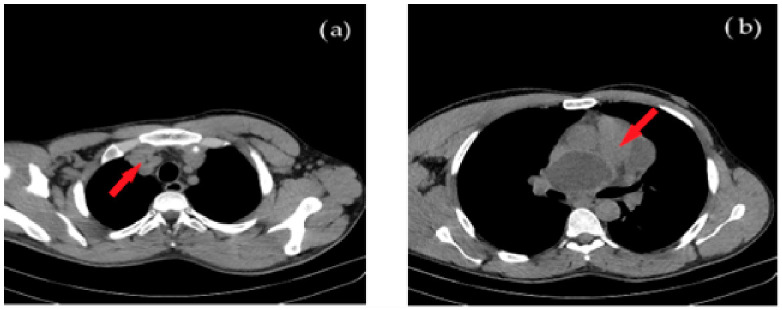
(a,b): Contrast and native CT of thorax and abdomen show multiple (a) mediastinal and (b) pericardial hydatic cysts.
